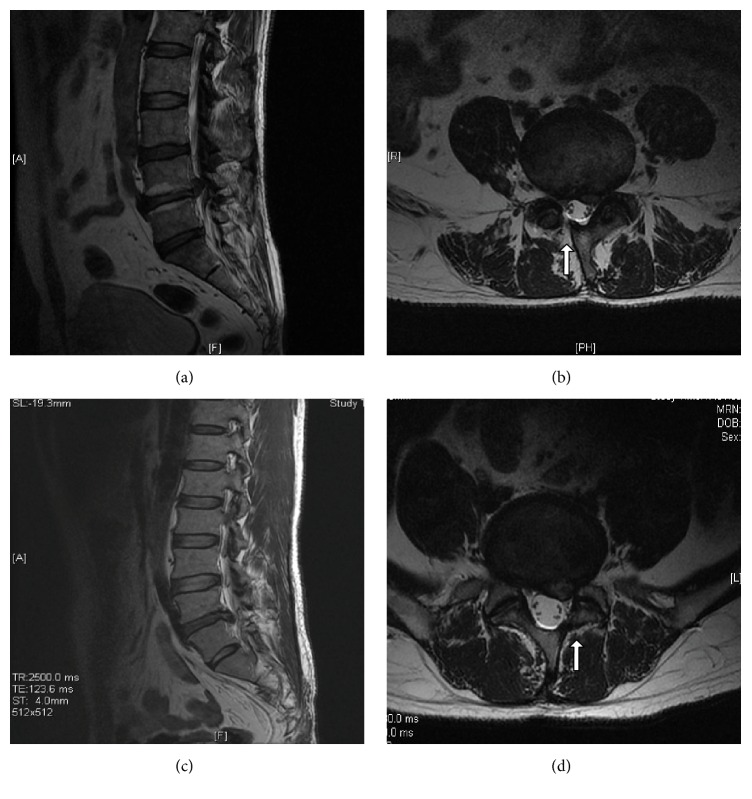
T2 sagittal (a) and axial (b) MRI show contralateral reherniation; T2 sagittal (c) and axial (d) MRI show ipsilateral reherniation. The arrow reflecting the laminectomy defect due to previous surgery.
